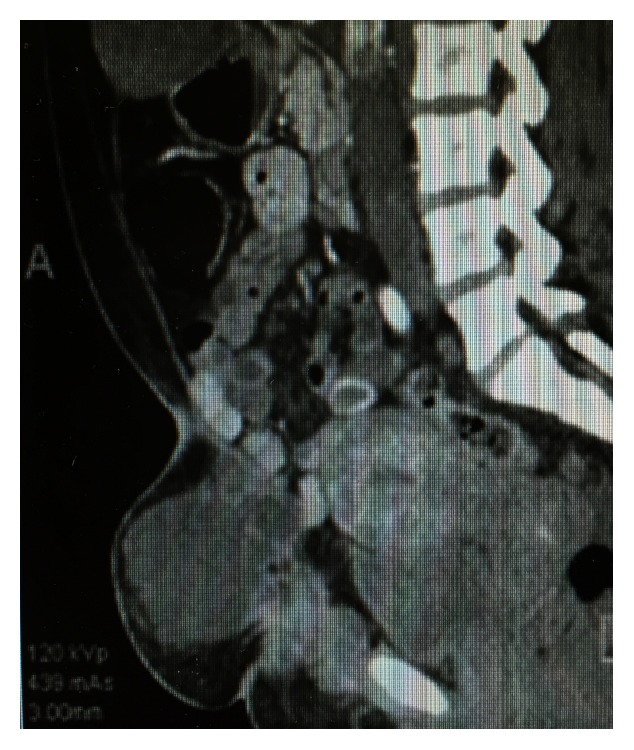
CT abdomen and pelvis demonstrating abdominal mass.
